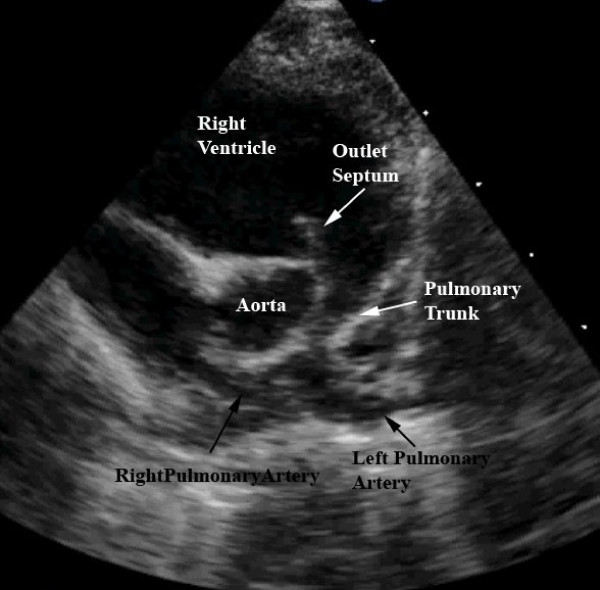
This still frame image of a parasternal short axis view of the echocardiogram of a patient with tetralogy of Fallot demonstrates the antero-cephalad deviation of the outlet septum into the right ventricular outflow tract.
